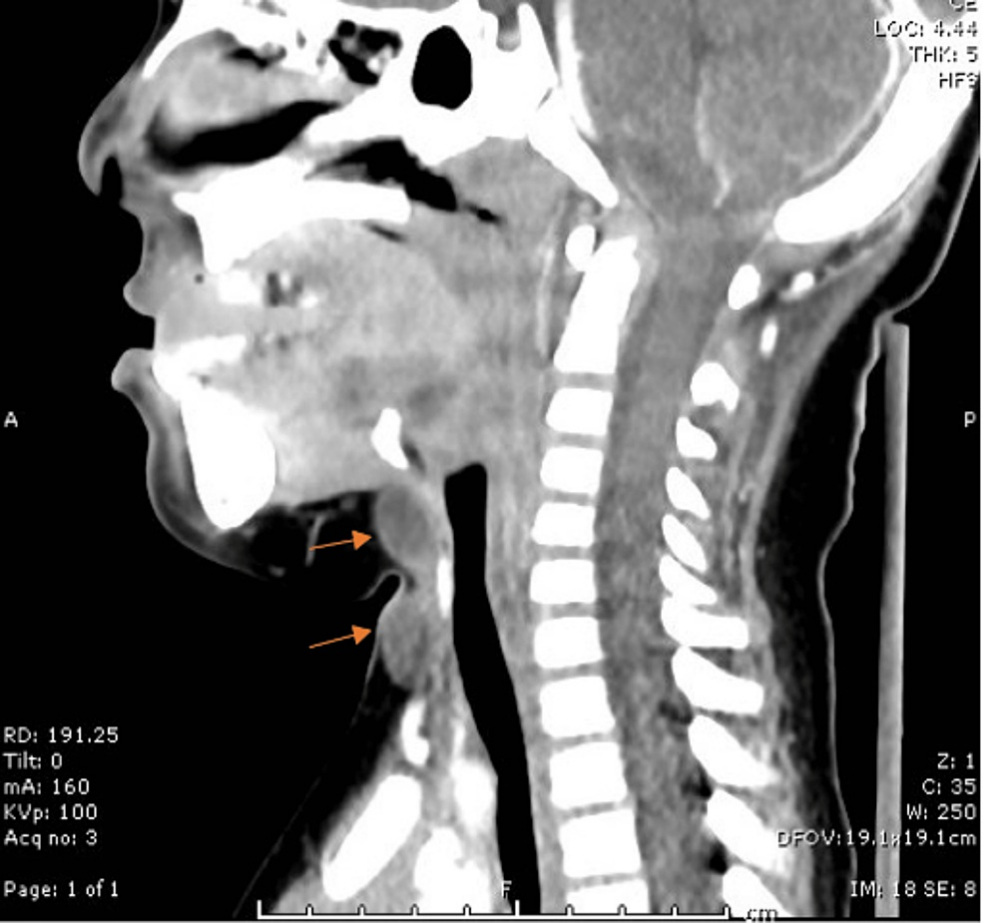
CT scan reveals two, well-defined smooth-bordered oval homogeneously appearing lesions located inferior to the hyoid bone and thyroid gland.
